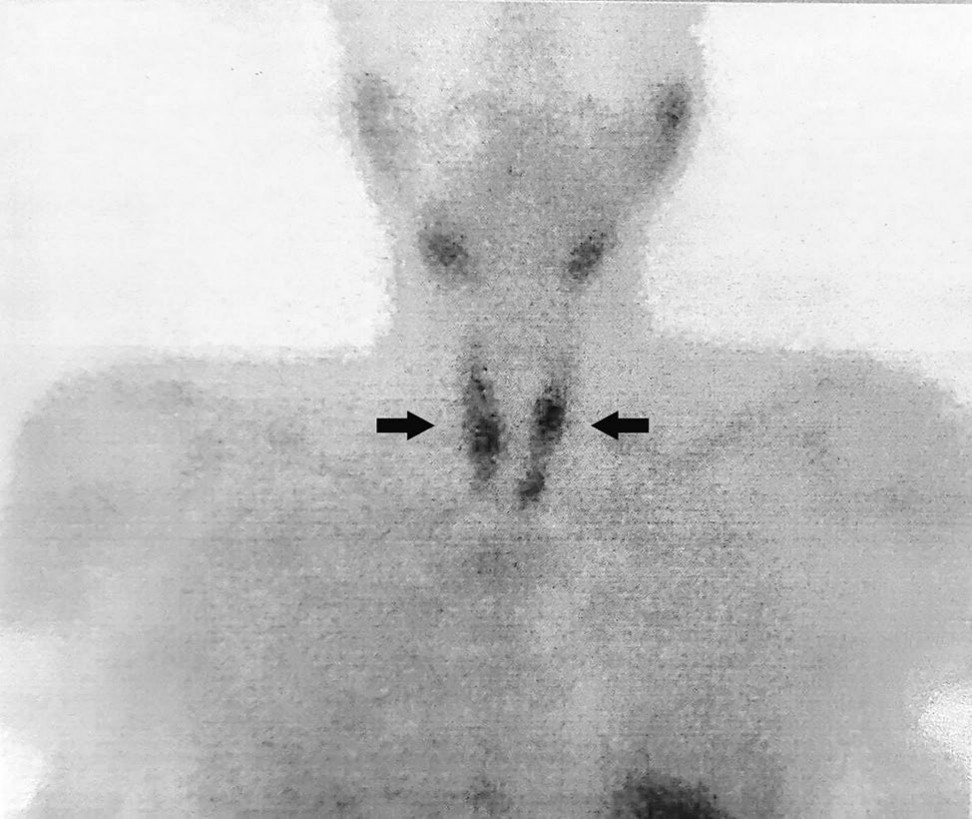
Sestamibi scintigraphy demonstrates increased uptake in all parathyroid glands, suggesting multiple parathyroid adenomas
